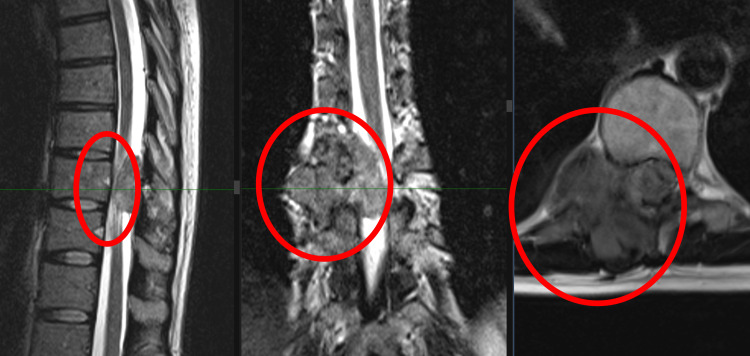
Preoperative MRI of the thoracic spine (2023), including sagittal and coronal T2-weighted sequences and axial T1-weighted post-contrast images, demonstrating a solitary metastatic lesion of the T10 vertebral body.
